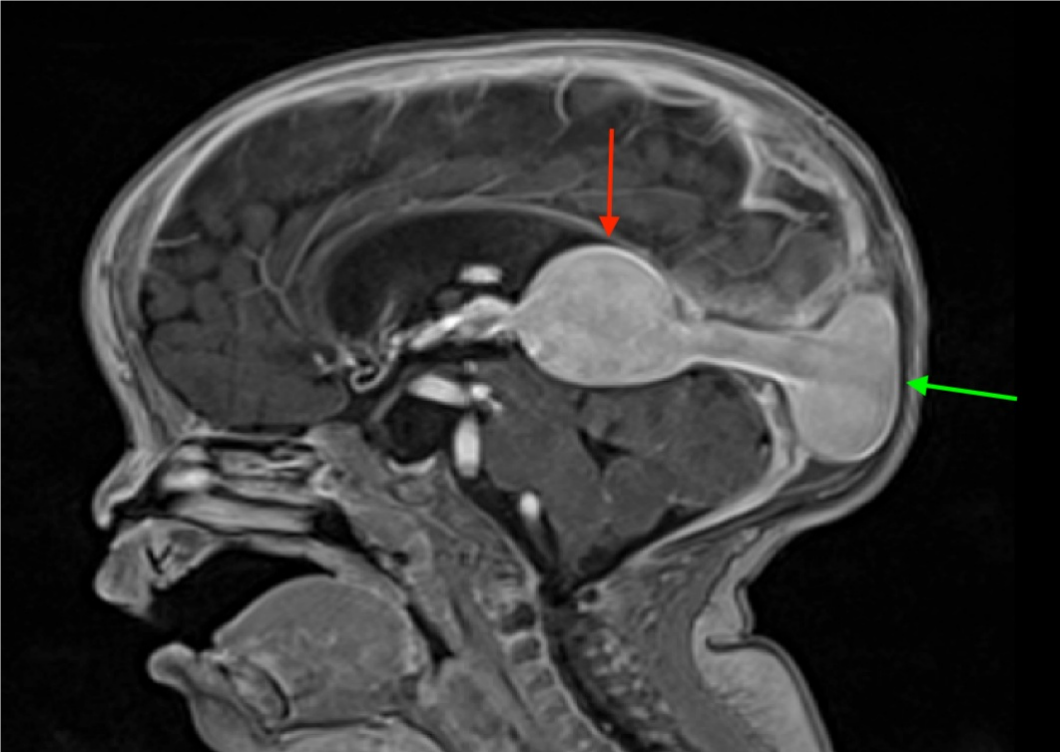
Sagittal T1-weighted MRI of a child with a VGAM (red arrow) Also note the enlarged torcular herophili (TH) (green arrow). MRI: magnetic resonance imaging; VGAM: vein of Galen aneurysmal malformation.
